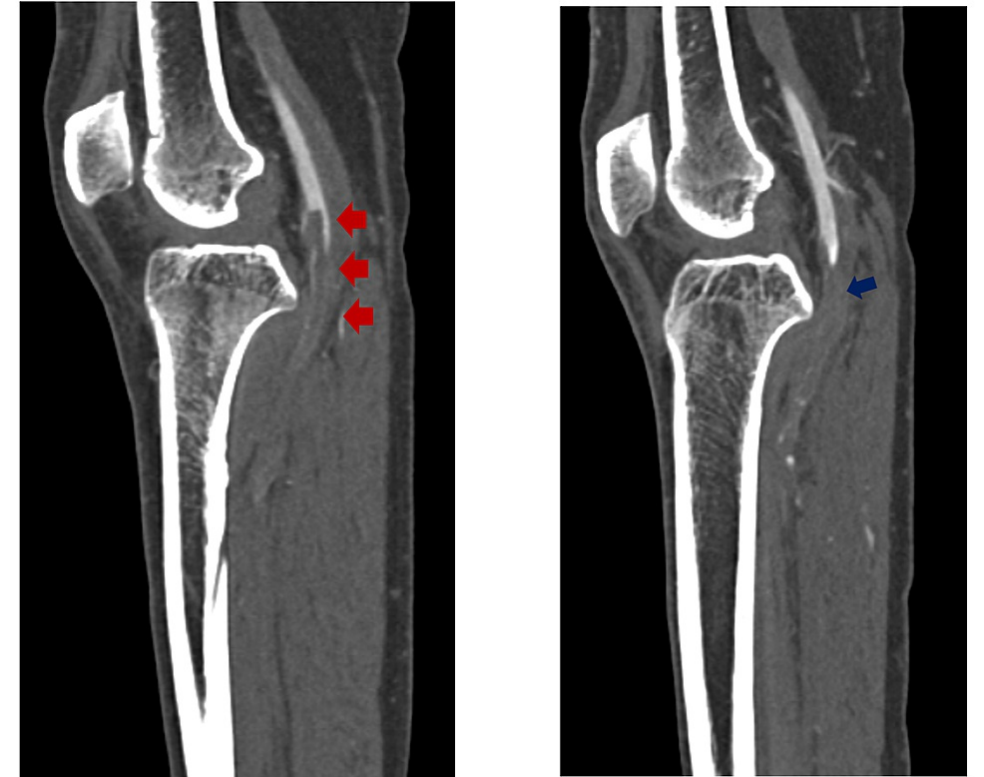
These are sagittal computed tomography angiography of both lower limbs, demonstrating complete occlusion of the right popliteal artery with downward thrombus extension to the anterior tibial artery (red arrow). Complete occlusion of the left popliteal artery thrombus with also downward extension of the thrombus to the anterior tibial artery (blue arrow)
